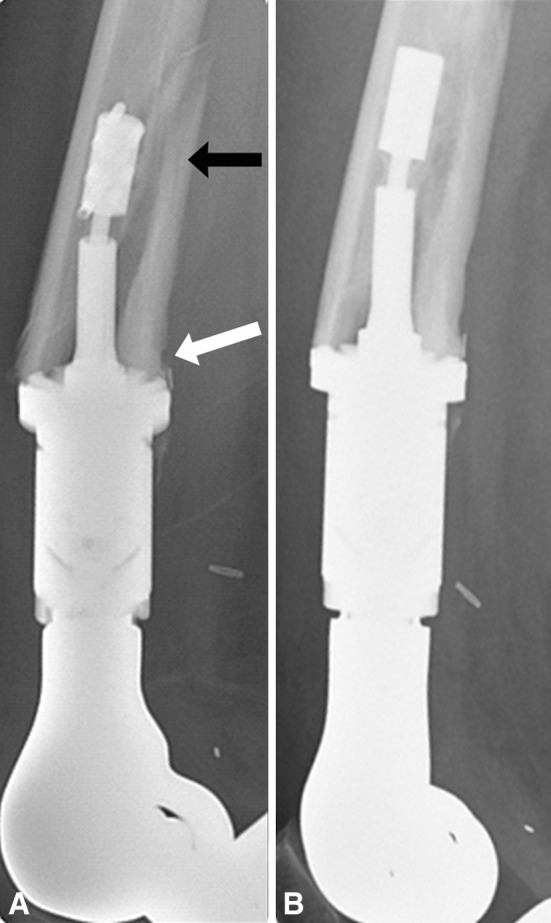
(A, B) Lateral radiographs taken 3 months apart show a Type IIB failure. (A) The black arrow points to the fracture of the posterior cortical segment that has moved with the spindle and the megaprosthesis, and the white arrow points to the intact bone-spindle interface where there has been some bone hypertrophy. Notably, the anterior cortex has not integrated and there is no hypertrophy. (B) The fracture healed spontaneously.
